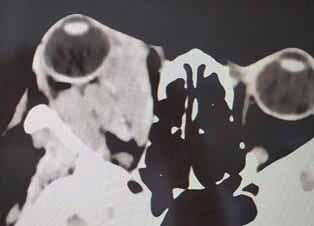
Magnetic resonance imaging showing retro-orbital and inferior-medial tumor mass.
